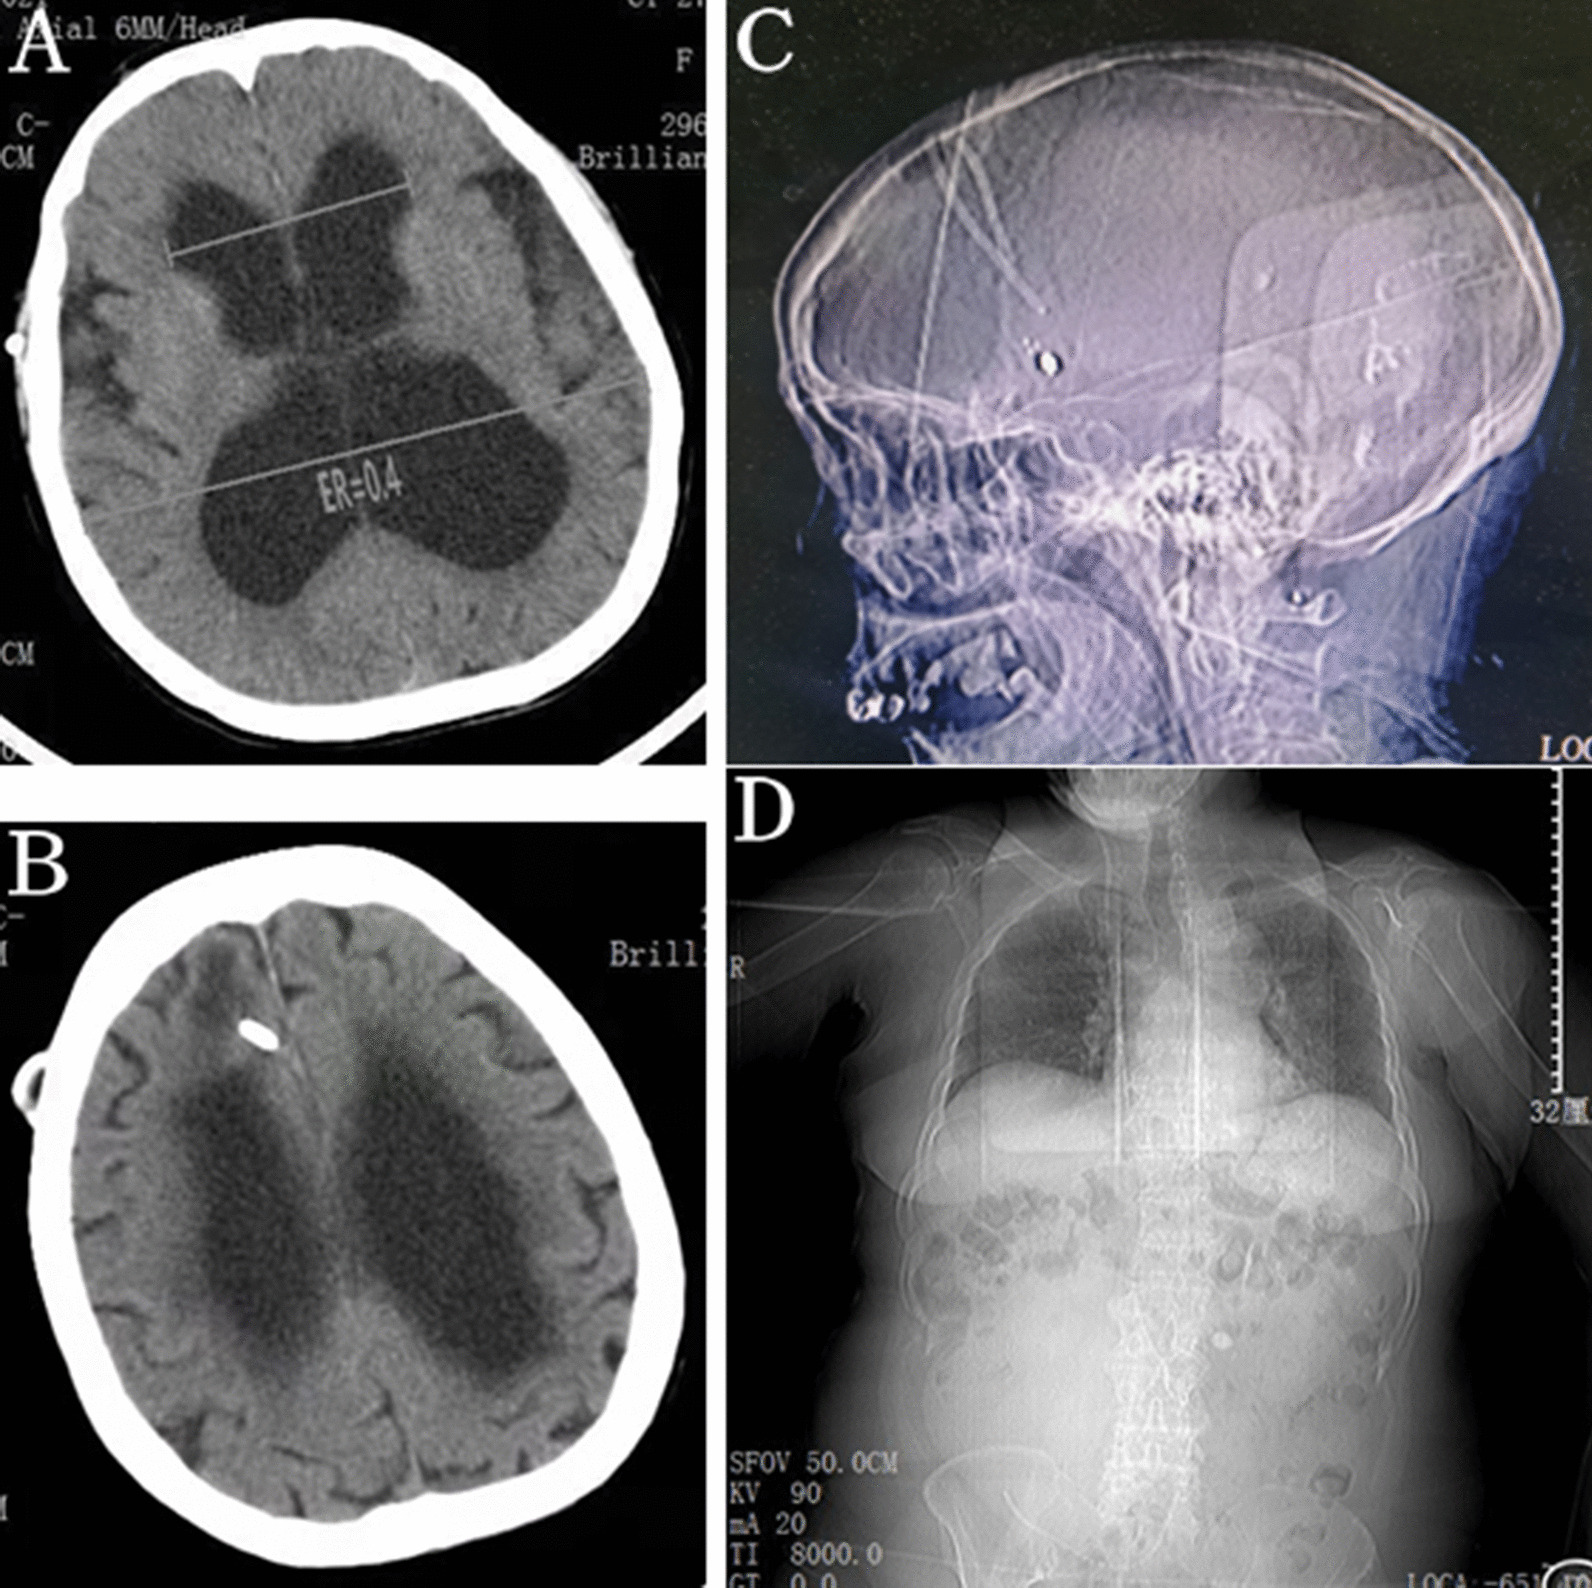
a Cerebral CT showed an enlarged ventricular system and its Evans Ratio was 0.4. b There being no interstitial cerebral edema and hemorrhage in brain or along the catheter. c, d The trajectory of the shunt on the skull and abdomen–thorax X ray did not show the catheter breakage, disconnection or migration
